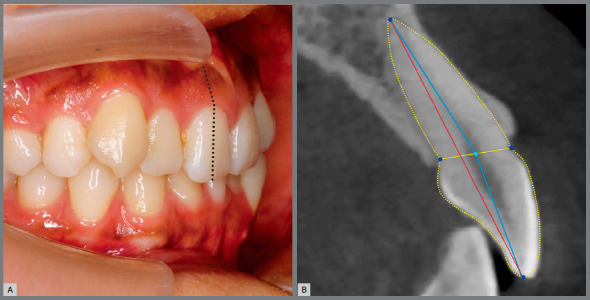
A) The intraoral photo potentially indicated the crown-root angulation phenomenon for the inclined root and upright crown in maxillary central incisor. B) Further lateral radiography confirmed that the long axes of crown and root didn’t coincide with each other, or the connection between incisor edge and root apex (red line) didn’t pass through the mid-point of the labial and lingual cementoenamel junction (yellow line).
